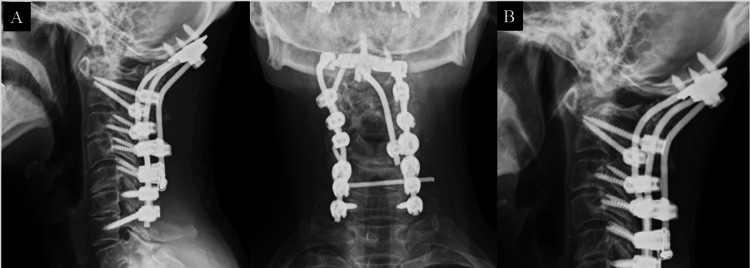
Revision surgery in case 2 (A) X-ray image after rod exchange with triple rod connection. (B) X-ray image obtained six months after revision surgery shows bony fusion without implant failure.
